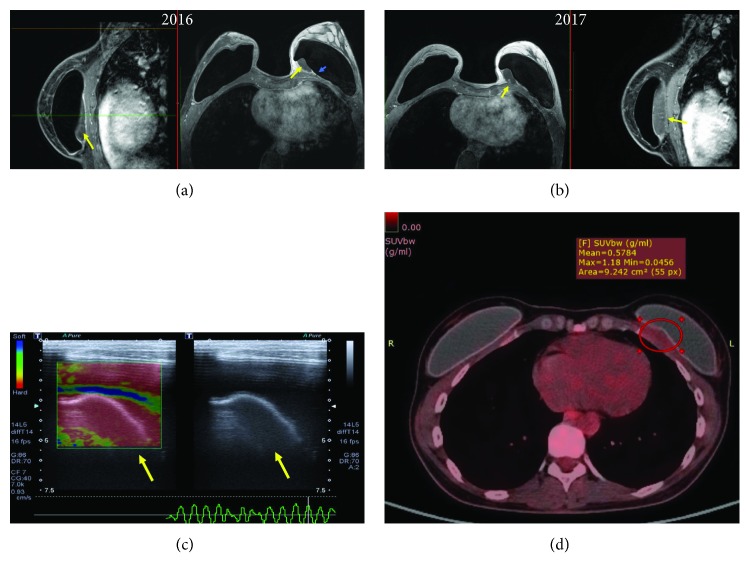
Intracapsular SIGBIC: imaging findings in a 40-year-old woman with left breast stiffening. (a) Breast MRI. The contrast BMRI shows an intracapsular mass with a slight contrast enhancement at coronal and axial images. Yellow arrows correspond to SIGBIC. Blue arrow corresponds to the black-drop signal. (b) One year after the first scan, the patient presented an increase of mass volume and of contrast enhancement. Yellow arrows correspond to SIGBIC. (c) Ultrasound. Intracapsular mass, posterior to the implant, with snowstorm-type aspect (yellow arrows). By elastography, it represents a hard lesion (left image). (d) PET scan. No significant radioisotope uptake by the mass (red circle) is observed. The radiological differential diagnosis is late seroma.
